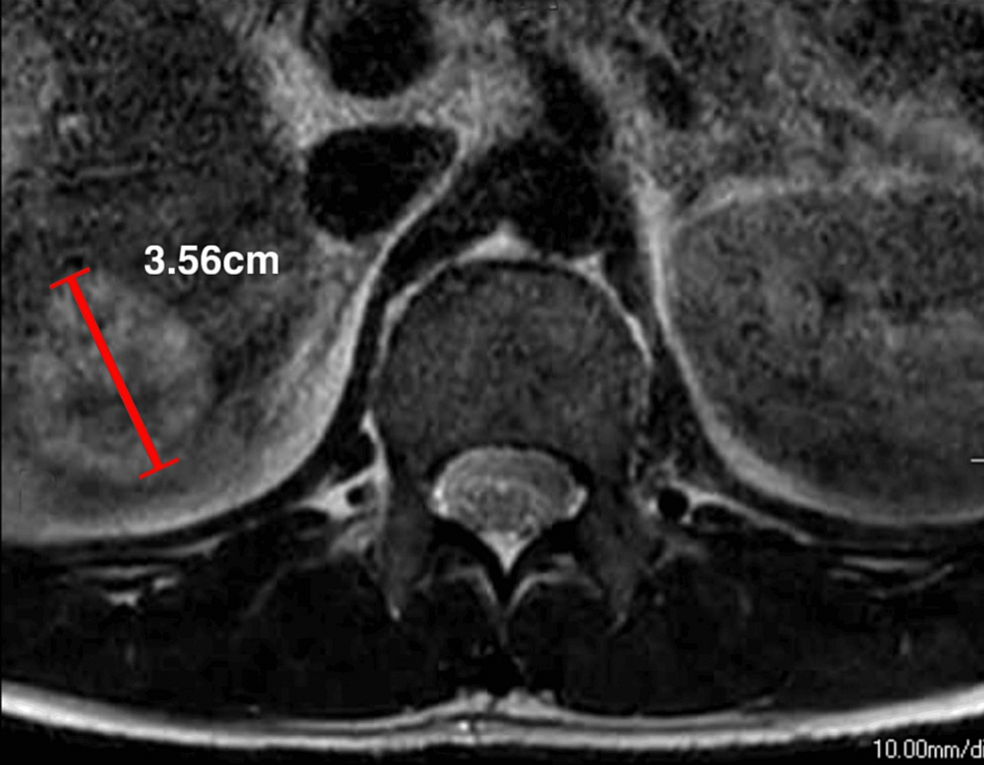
Axial view of magnetic resonance image (MRI) of lumbar spine Multiple liver lesions measuring up to 3.56 cm are seen, indicative of liver metastases.
